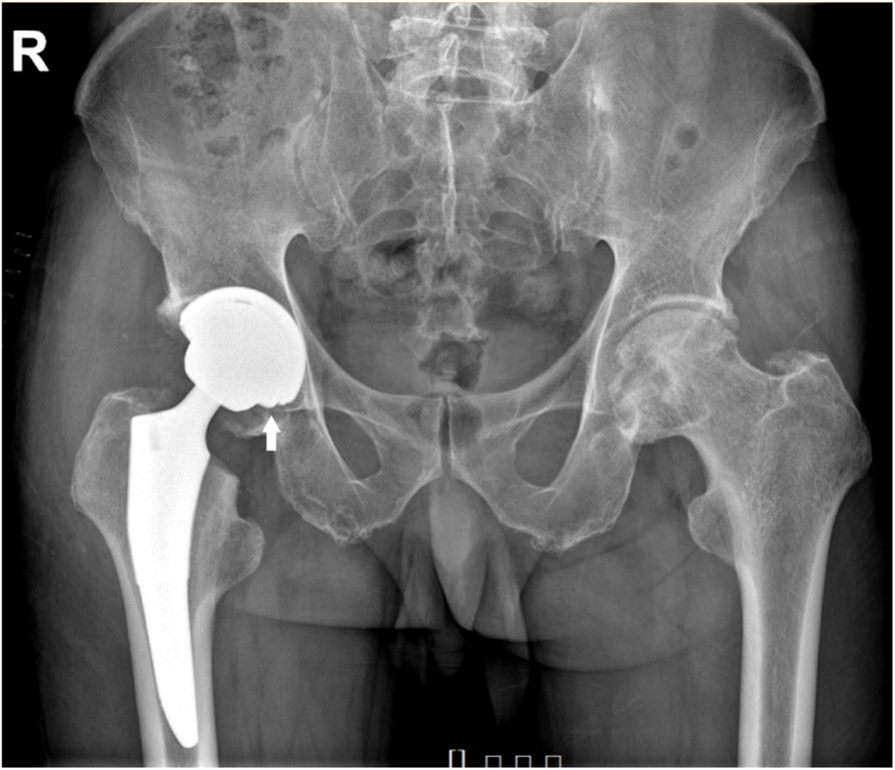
Plain radiograph on the eighth day after the surgery.Ceramic liner dissociation can be observed on a plain hip radiograph (arrow), which is almost identical with the presentation on the radiograph of the first postoperative day (Fig. 2a)
